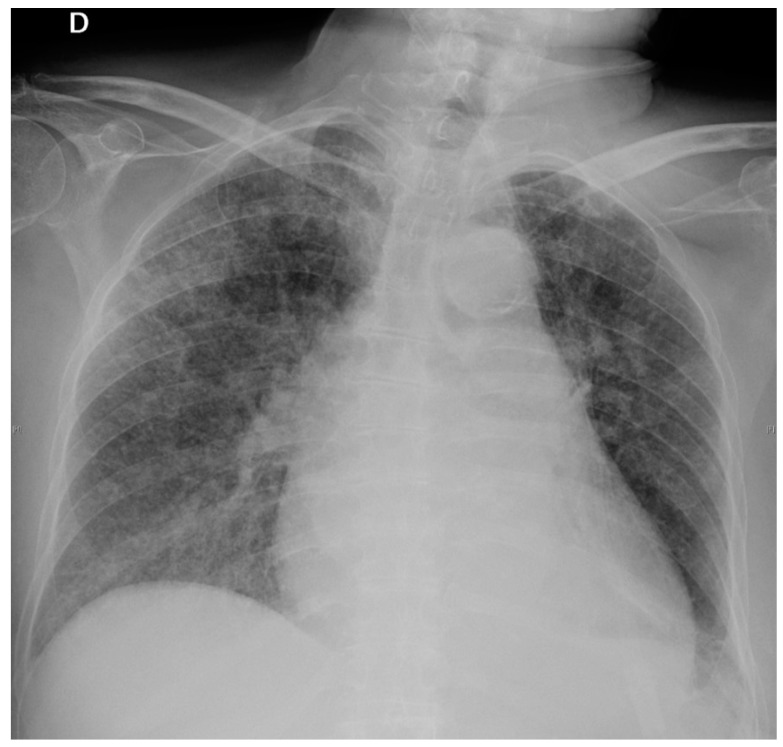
Chest X-ray on 7th day: Bilateral interstitial infiltrates.
